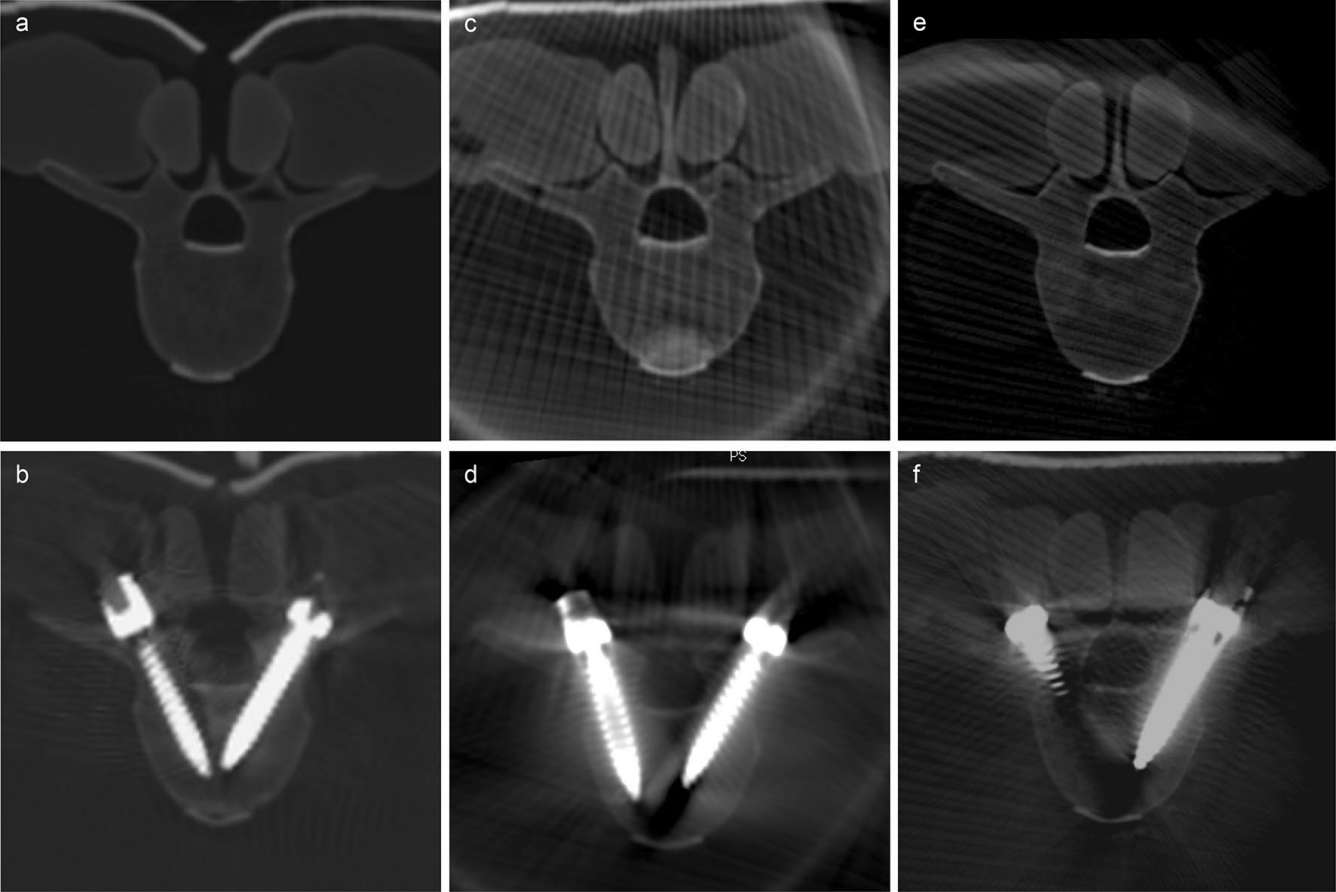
Visualization of pedicles before (a,c,e) and after screw placement (b,d,f) in the lumbar spine (L3) in intraoperative imaging. (a/b) iCT, (c/d) C-arm CBCT, (e/f) CBCT.
